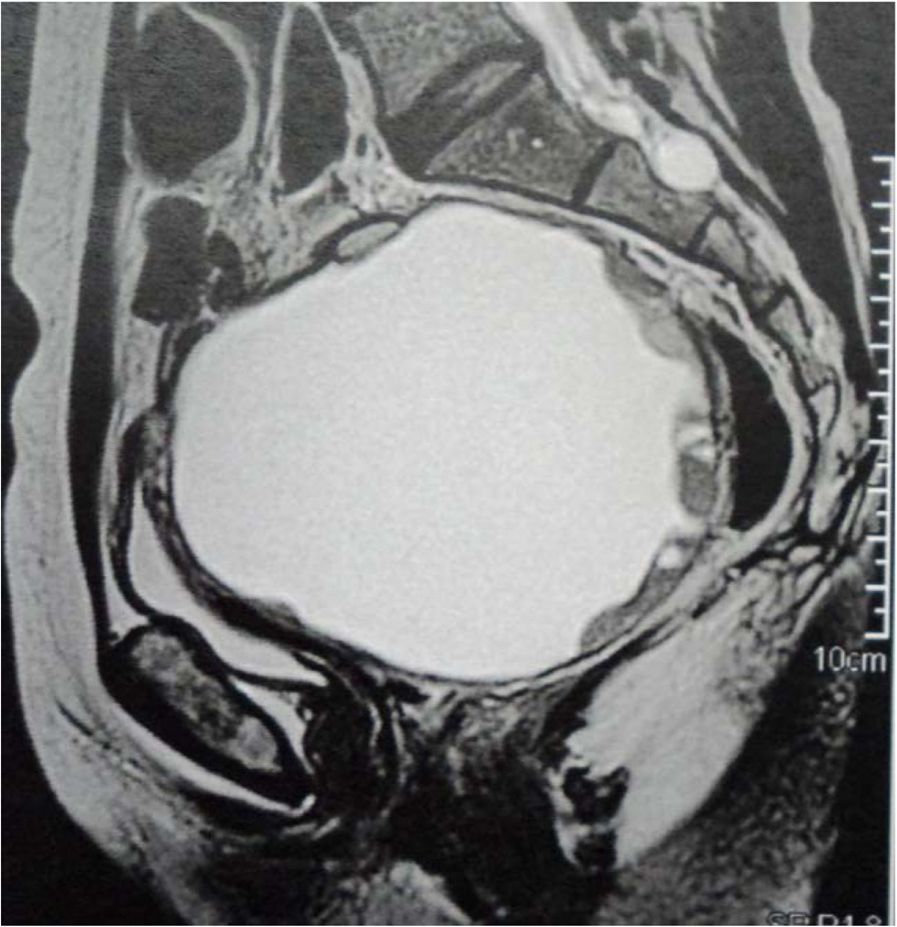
Sagittal T2 weighted pelvic MRI images
